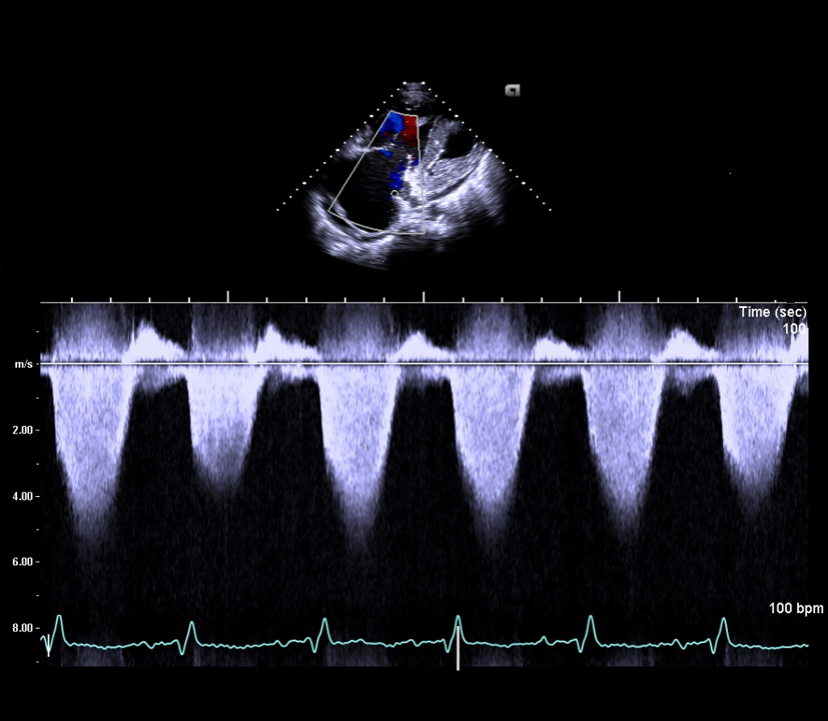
Continuous wave doppler signal showed the peak tricuspid valvular regurgitation velocity was 4.2 m/sec and peak pressure gradient was estimated at 70.56 mmHg.
